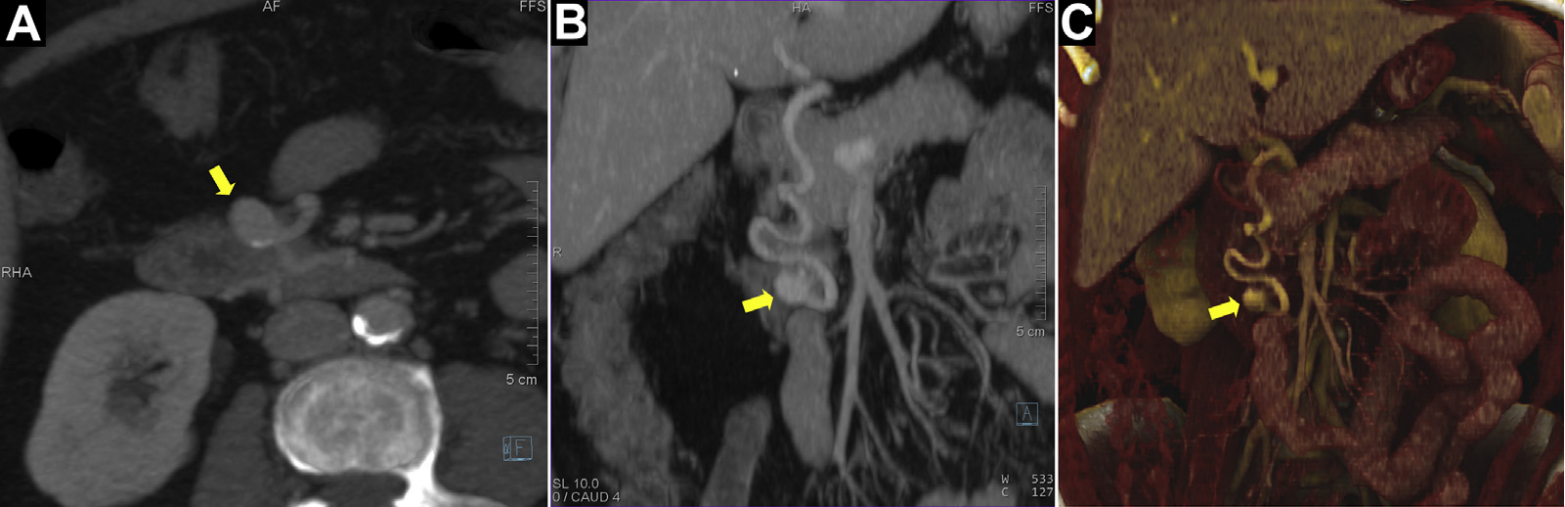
Axial (A), coronal (B), and volume rendered (C) reconstructions of preoperative computed tomography angiography (CTA) images demonstrating a 1.7- × 1.1-cm aneurysm involving the anterior pancreaticoduodenal artery (PDA; *yellow arrow*).
